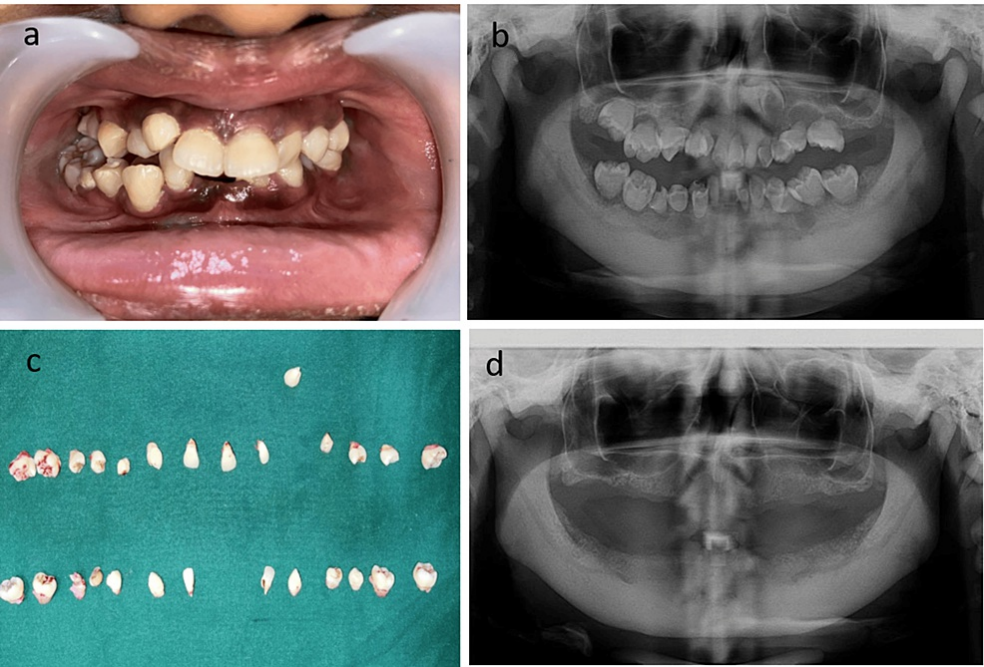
a) Intraoral photograph showing dentition with dentin dysplasia. b) Pre-operative orthopantomogram showing the rootless teeth appearance of all teeth. c) Extraction of all teeth performed. d) Post-extraction radiograph showing complete edentulous space
